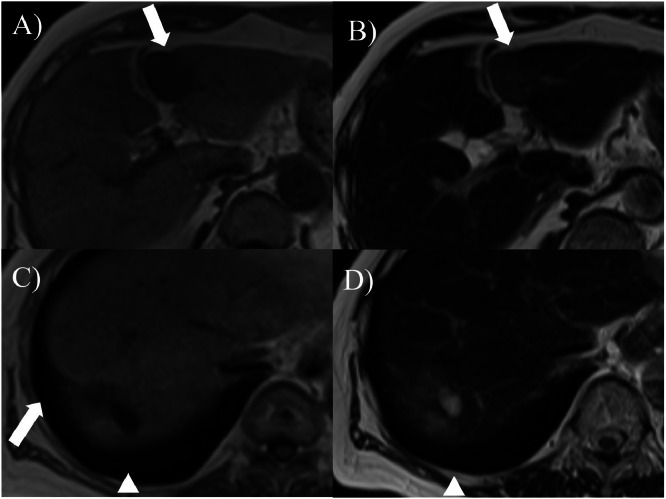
Dynamic abdominal magnetic resonance imaging findings (2001+23 years). Atrophic masses measuring 28×21 mm in liver S2/3 (A, B) and 21×20 mm in liver S8 (C, D) are observed. T1WI shows low signal intensity (A, C), and T2WI shows faint high signal intensity (B, D). A concave deformation of the liver surface is observed (arrow). The cyst near S8 has expanded from 9 to 20 mm. (arrowhead).
